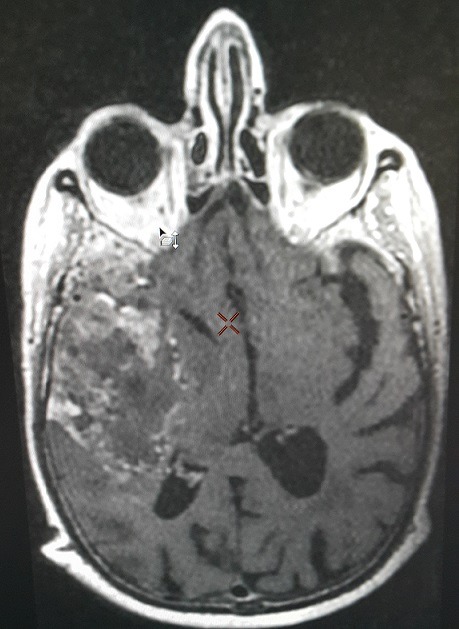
Brain MRI (axial section, injected T1-weighted sequence) showing a huge right temporal mass, taking contrast heterogeneously, with a shift of the midline
